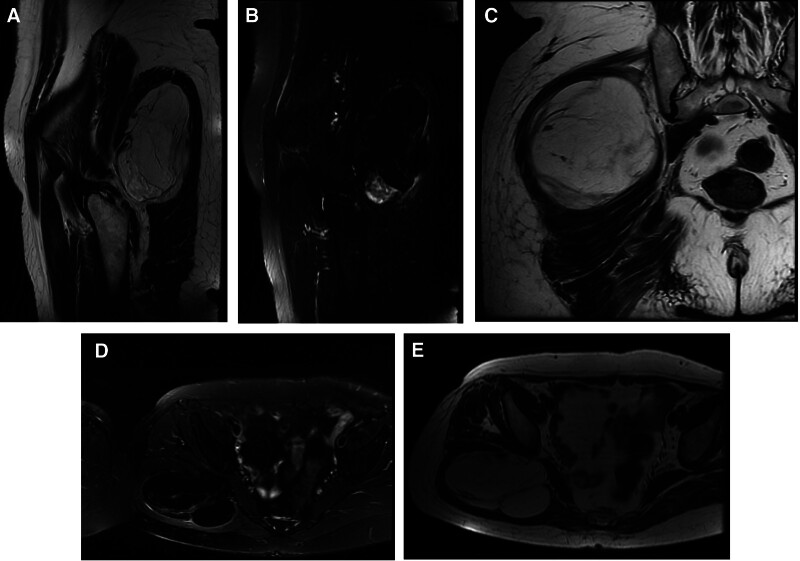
(A–E) MRI. On MRI (T1/T2-weighted sequences in coronal, sagittal, and axial planes), a well-defined gluteal mass measuring 10.37 × 10.55 × 6.2 cm (LR × SI × AP) was identified, demonstrating compression of neighboring muscular soft tissue structures. AP = anterior–posterior, LR = left–right, MRI = magnetic resonance imaging, SI = superior–inferior.
